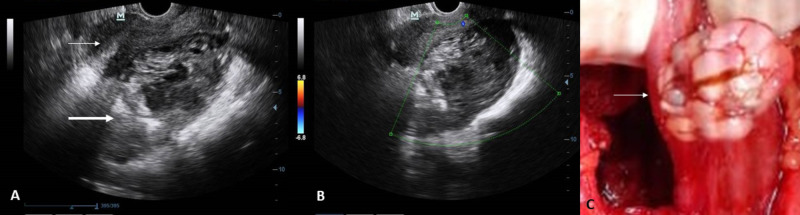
Ultrasound and intraoperative image (A) Two-dimensional ultrasonography showing a normal-sized uterus (thin arrow) with a well-defined heterogeneous lesion in the pouch of Douglas (POD) measuring 9.0 x 8.5 x 6.4 cm (thick arrow). (B) Color Doppler ultrasonography showing no internal vascularity in the lesion in the POD. (C) Intraoperative picture showing incidental finding of the left ovarian dermoid tumor (arrow).
